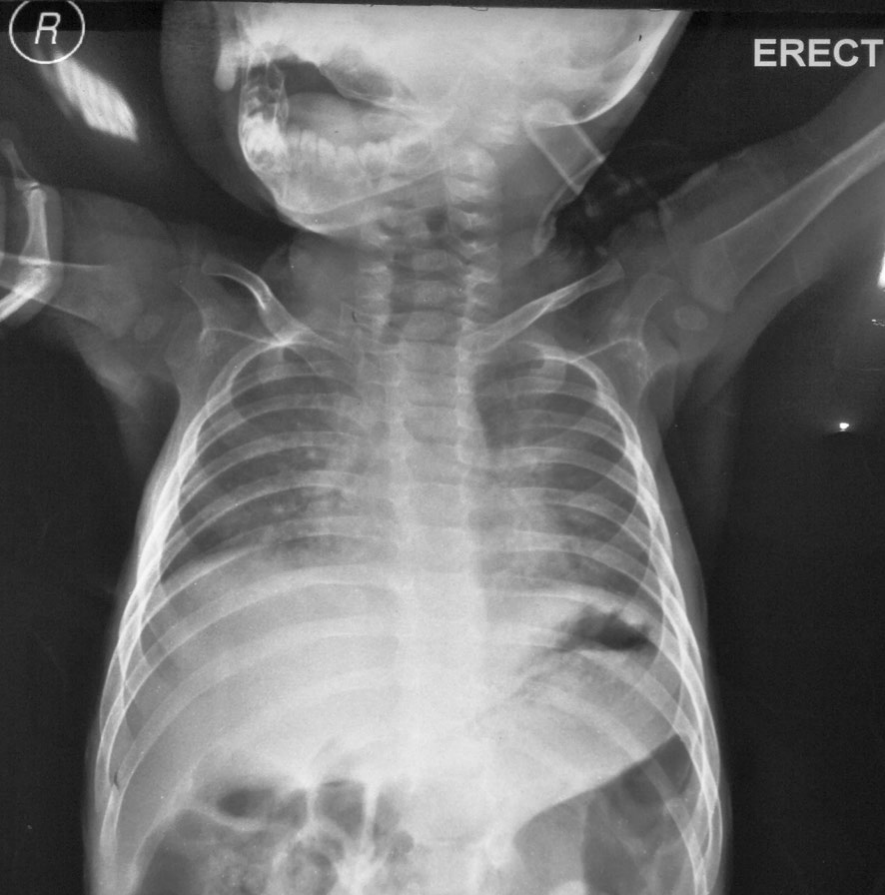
Plain chest X-ray film suggested cardiomegaly with increased interstitial markings and upper mediastinal lymph nodes enlargement.
